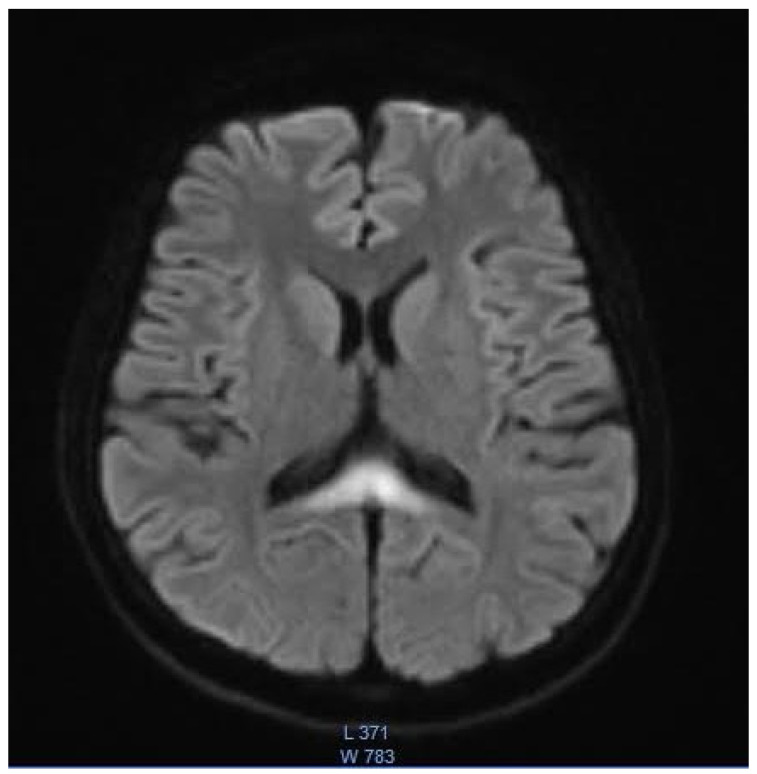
The DWI reveals a high-signal intensity within the splenium of the corpus callosum.
